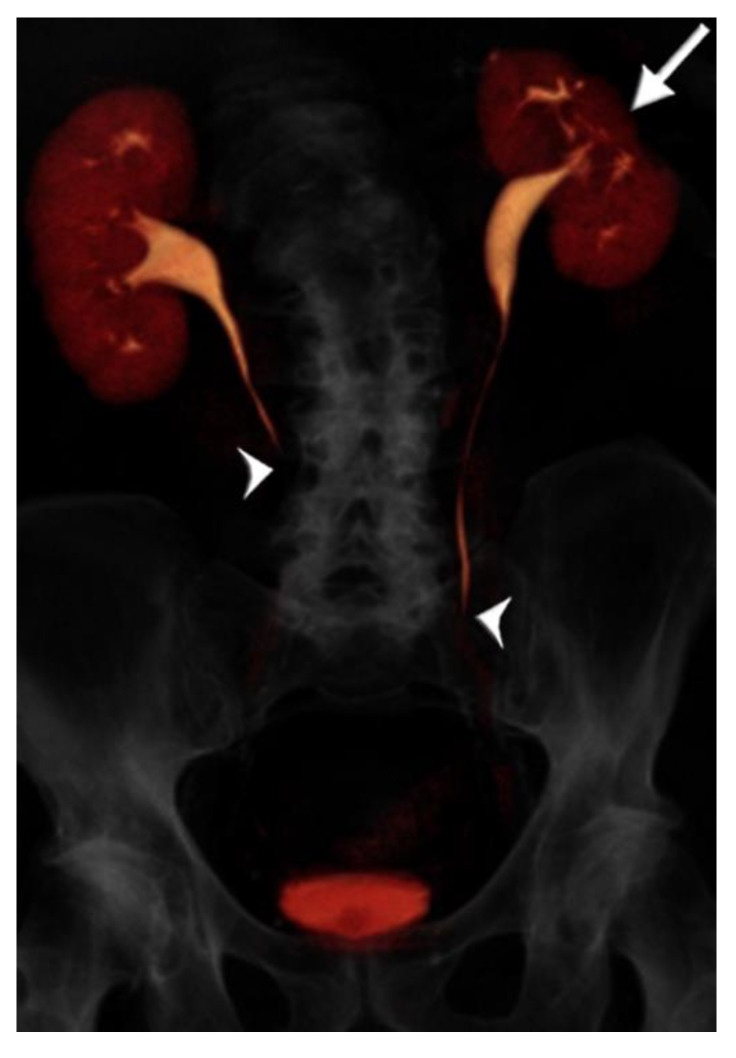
73 year-old male with microhematuria imaged with split-bolus CT urography. Volume-rendered image of the first excretory phase shows no contrast opacification of the mid-distal ureters (arrowheads). There is cortical scarring of the left kidney from previous nephrectomy for renal cell carcinoma (arrow). On repeat acquisition of the excretory phase, no improvement in image quality was achieved. The total table time was 17 min and the dose-length product was 1731 mGy·cm.
